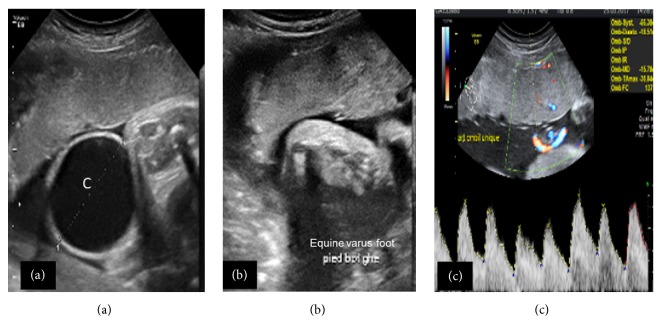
Morphological anomalies seen at antenatal ultrasound. (a) Cystic perineal mass (C), (b) equinovarus left foot, and (c) umbilical cord with a single artery.
